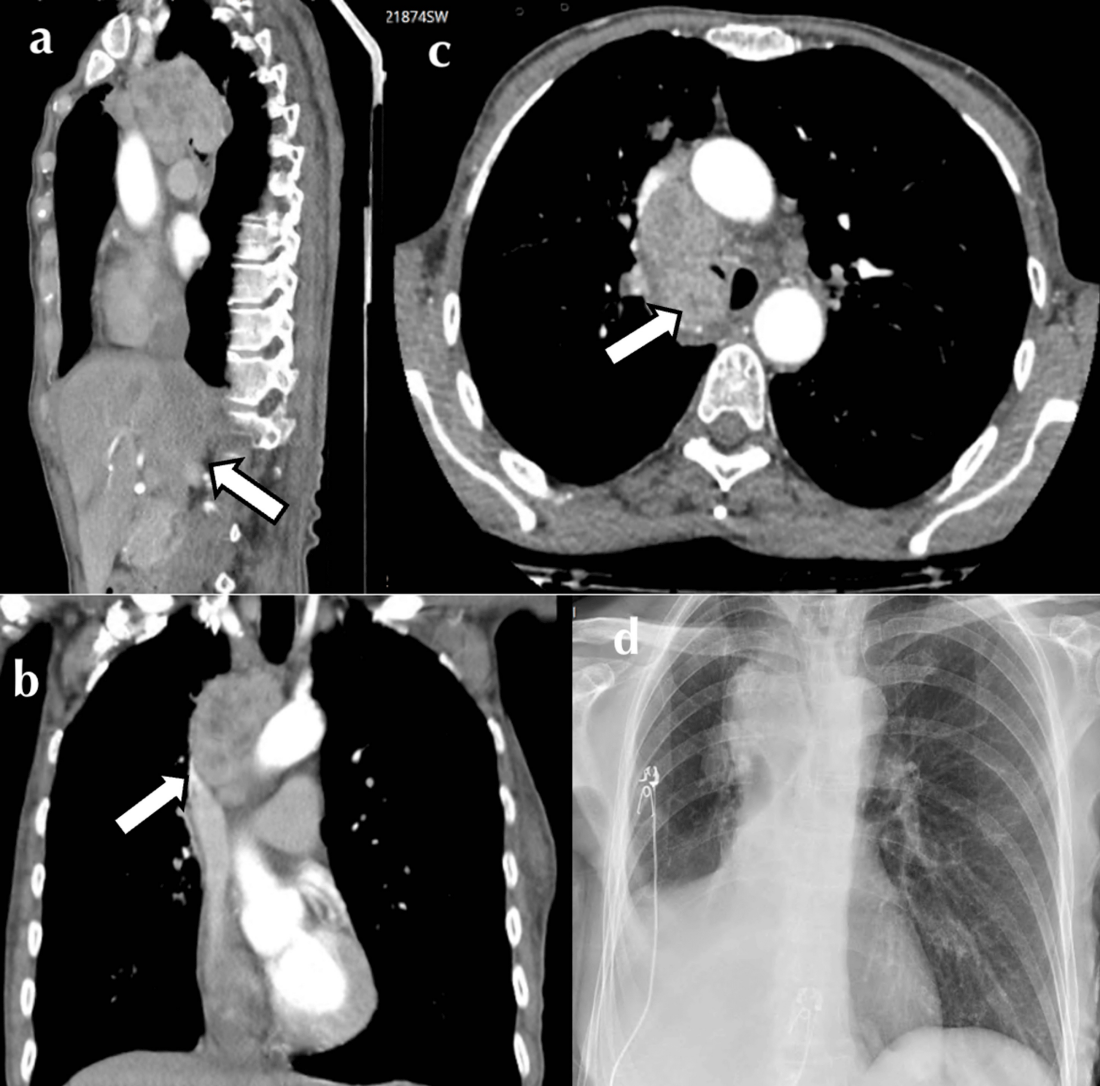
Computed tomography (CT) and chest X-ray (CXR) imaging demonstrating a large mixed-density mediastinal mass causing right main bronchus compression and right middle and lower lobe collapse: (a) sagittal, (b) coronal, (c) axial, and (d) AP-CXR.
